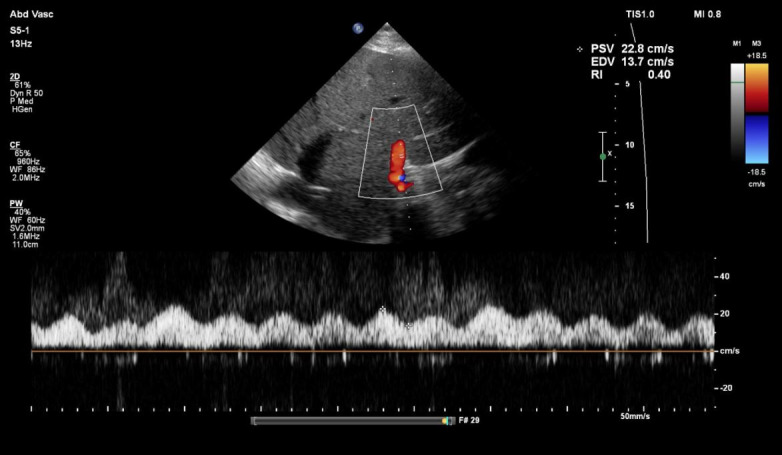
Portal vein Doppler demonstrating mildly increased pulsatility.
